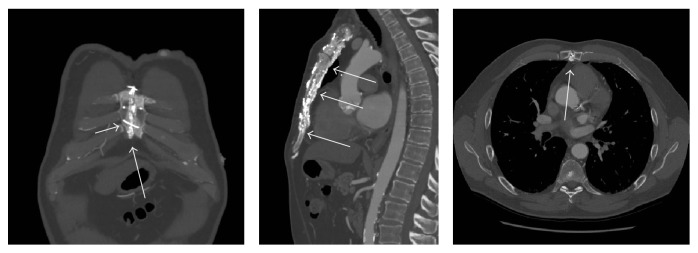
CT angiography 6 years post-CABG demonstrating sternal closure with wire cerclage (short arrow) and Kryptonite bone cement (long arrows).
